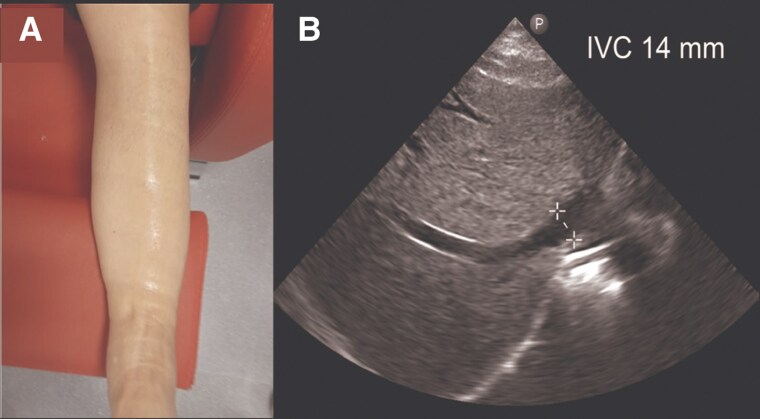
Redistribution of volume after 24 h of venous leg compression. (*A*) Reduced ankle circumference from 26 to 21 cm with residual mild oedema (I/IV). (*B*) Inferior vena cava echocardiography at 14 mm after 24 h of venous leg compression.
